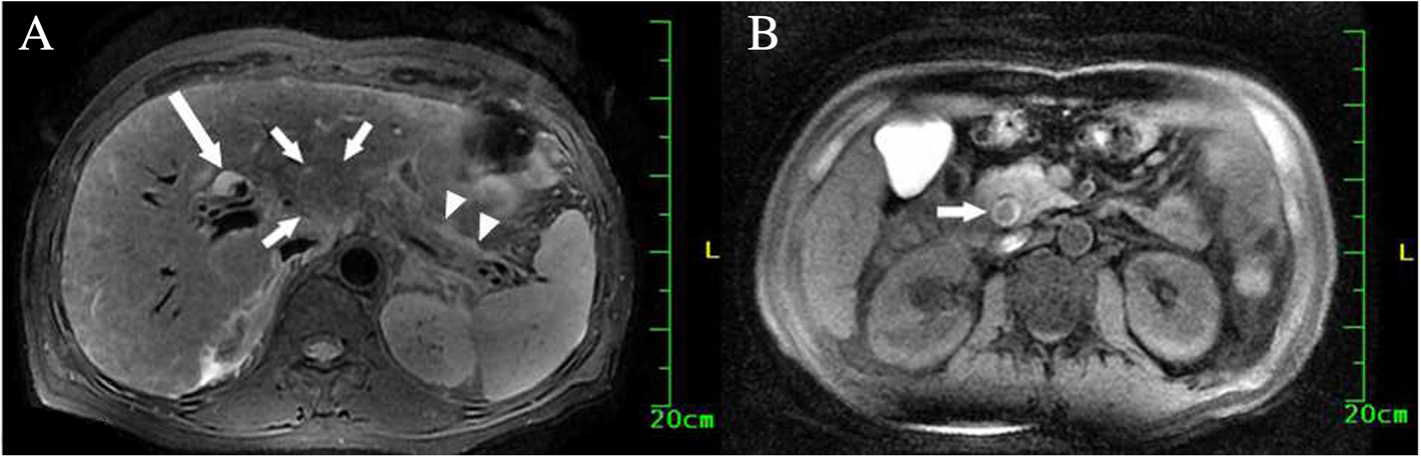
Magnetic resonance imaging findings on 21 September 2011. (A) Short inversion time inversion recovery image, showing an irregular, isointense mass with unclear margins (short white arrows) in the left lobe of the liver. There was fluid around the pancreas (white arrows) indicating pancreatitis, and mild dilation of the intrahepatic bile ducts (long white arrow). (B) Short inversion time inversion recovery image distal to the plane of image (A), showing a dilated common bile duct with an isointense filling defect (white arrow).
